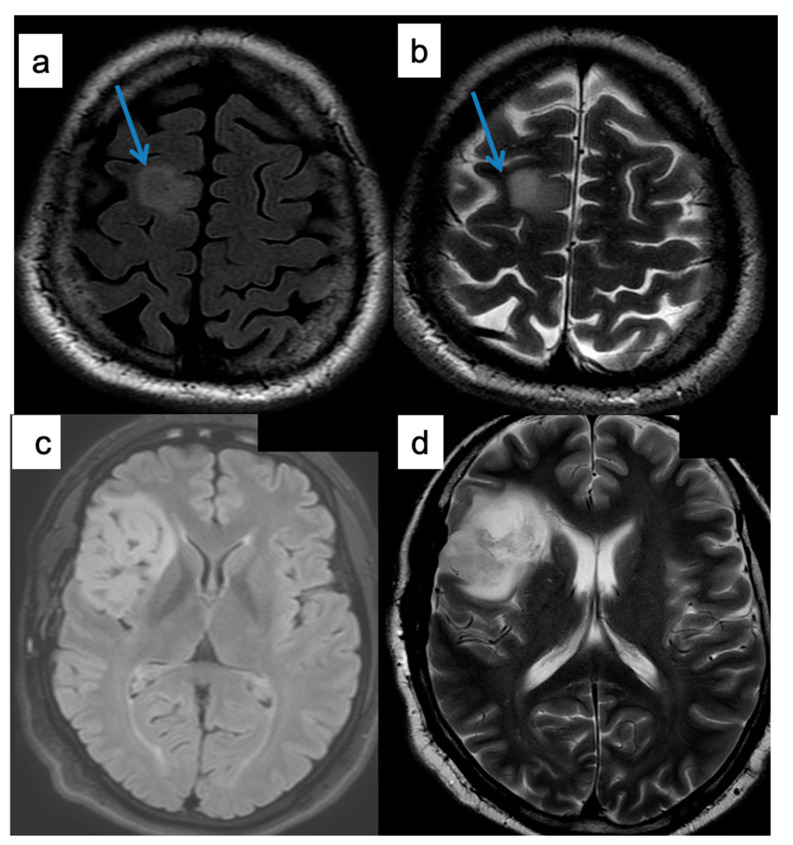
T2–FLAIR mismatch sign. (a,c) are fluid-attenuated inversion recovery (FLAIR) images while (b,d) are T2 weighted images (WI).
